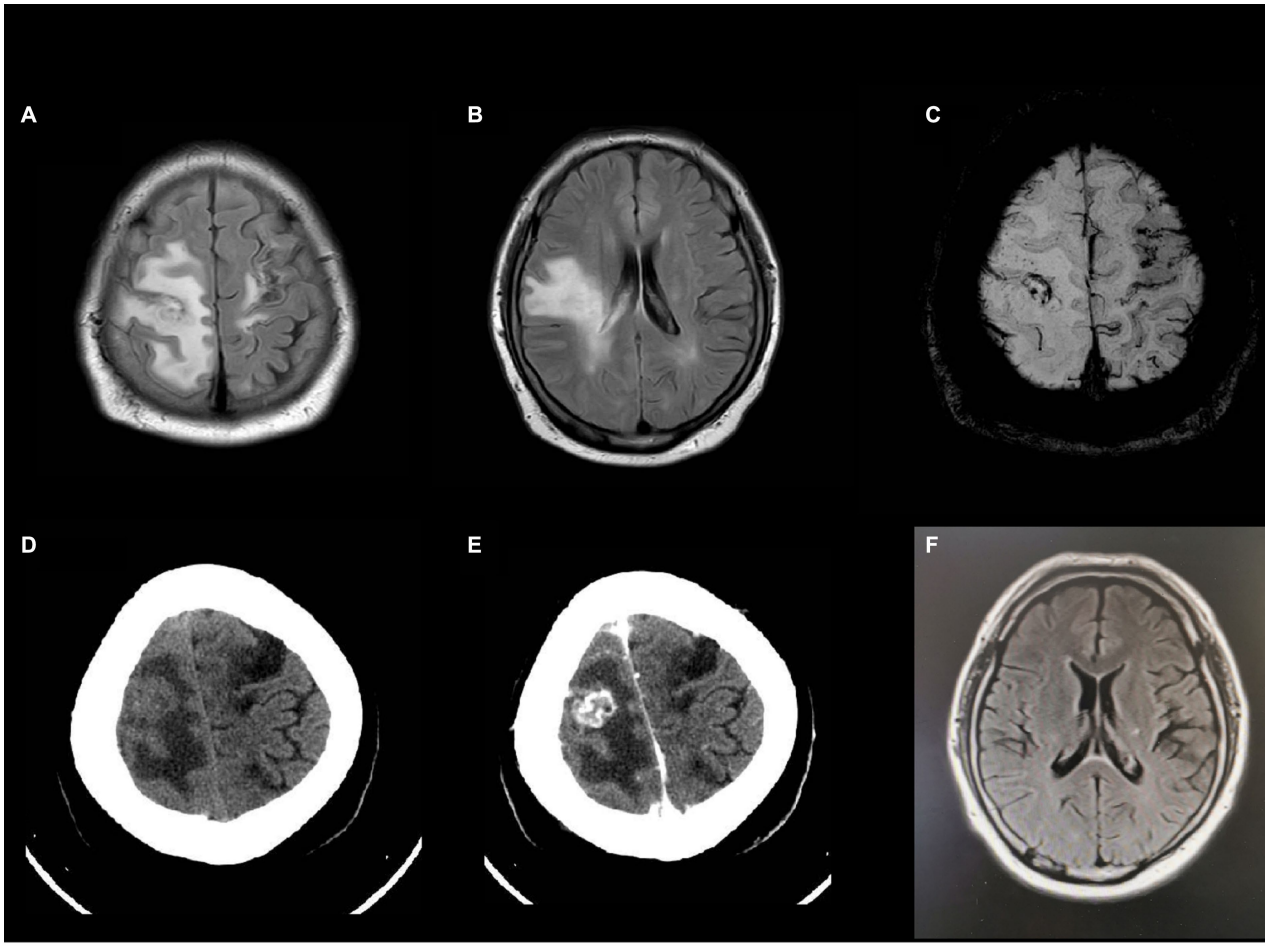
Head MRI and enhanced CT after admission (A,B) T2WI showed expansion of white matter hyperintensity with mixed signals inside. (C) Susceptibility weighted imaging (SWI) indicated microbleeds at both new lesions and old infarctions. (D,E) Hypodensity lesions in head CT scan and rim-like or nodule-like enhancement inside the lesion in enhanced CT. (F) Head MRI 18 months later showed that the extent of lesion had regressed significantly.
